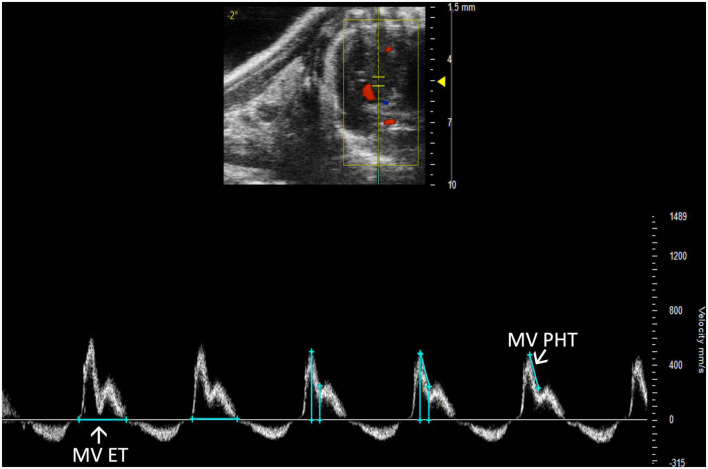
Pulsed wave (PW) Doppler mode image showing mitral valve pressure half time (MV PHT), a measure of mitral stenosis and mitral valve ejection time (MV ET), the duration of diastolic filling in the left ventricle.
